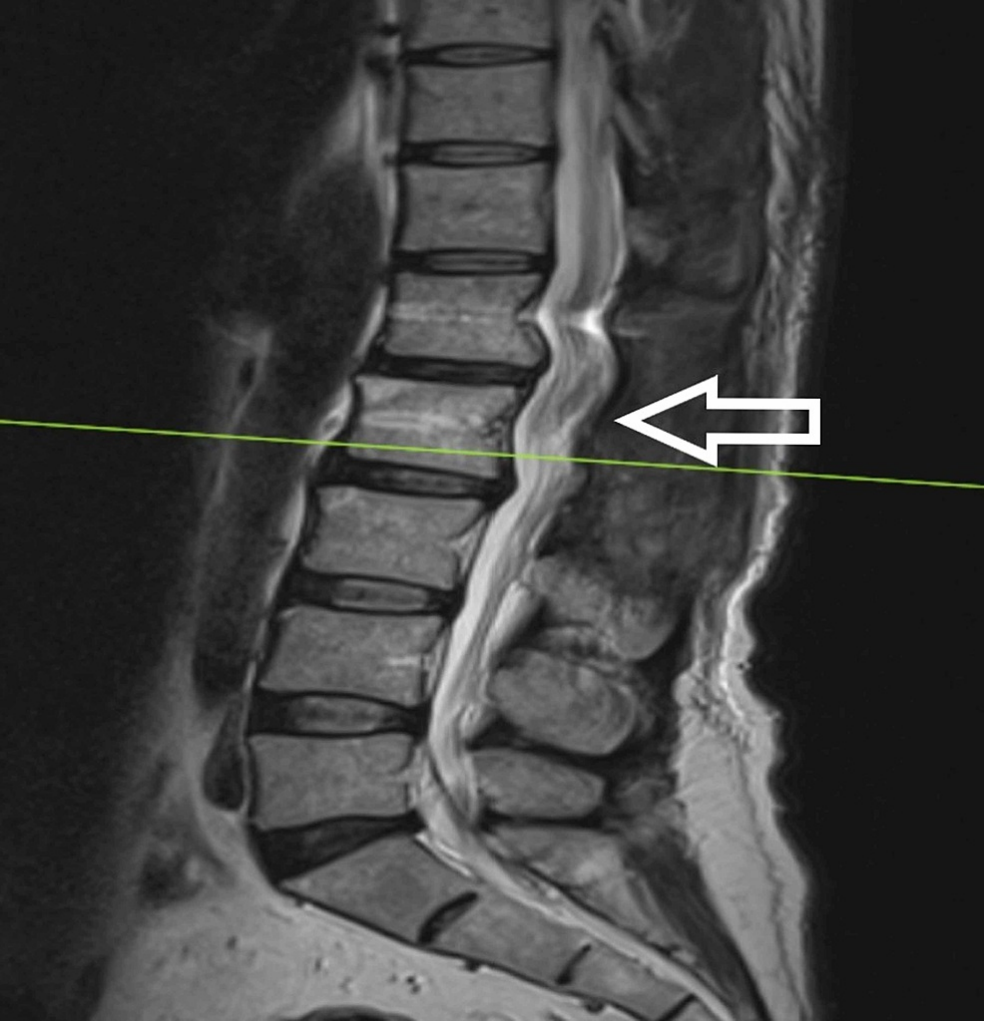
Immediate post-operative sagittal MRI
